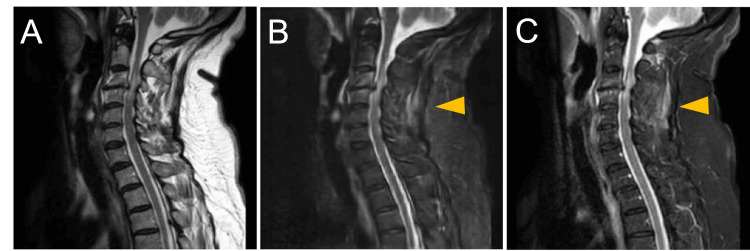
A representative case featuring images generated by a GAN (A) A T2-weighted image of a spinal cord injury: the interspinous ligament injury is not obvious; (B) A synthetic STIR image of SCI: the high-intensity changes are seen in the interspinous ligament (yellow arrowhead); (C) An actual STIR image of SCI: the high-intensity changes are seen in the interspinous ligament (yellow arrowhead). SCI: spinal cord injury; STIR: short T1 inversion recovery
